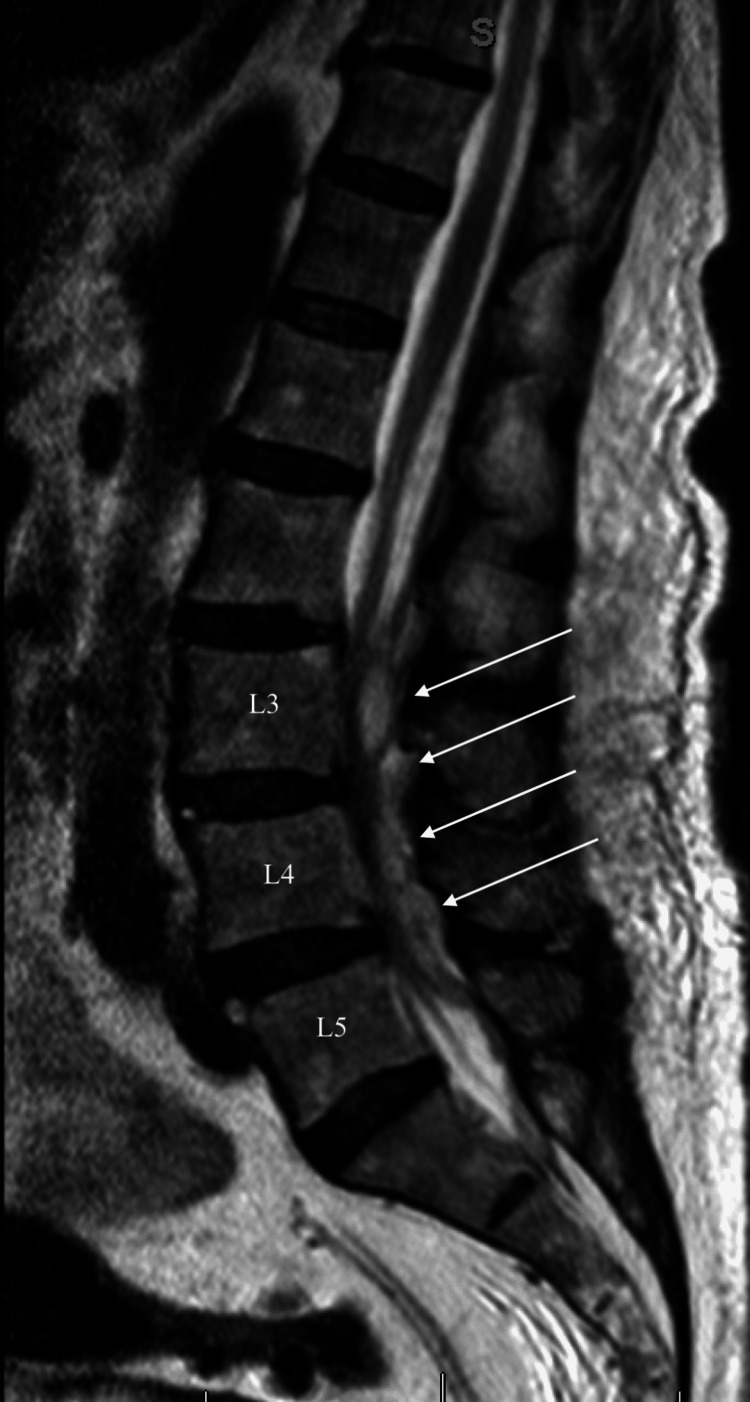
T2-weighted sagittal MRI of the lumbar spine without contrast Neurological exam findings: cranial nerves 2-12 intact without facial asymmetry. Upper extremity strength 5/5, lower extremity strength 3/5, deep tendon reflexes +2 in all extremities, no saddle anesthesia, no Hoffmann sign, no dysdiadochokinesia, and no clonus. Positive straight leg raising on the right.
